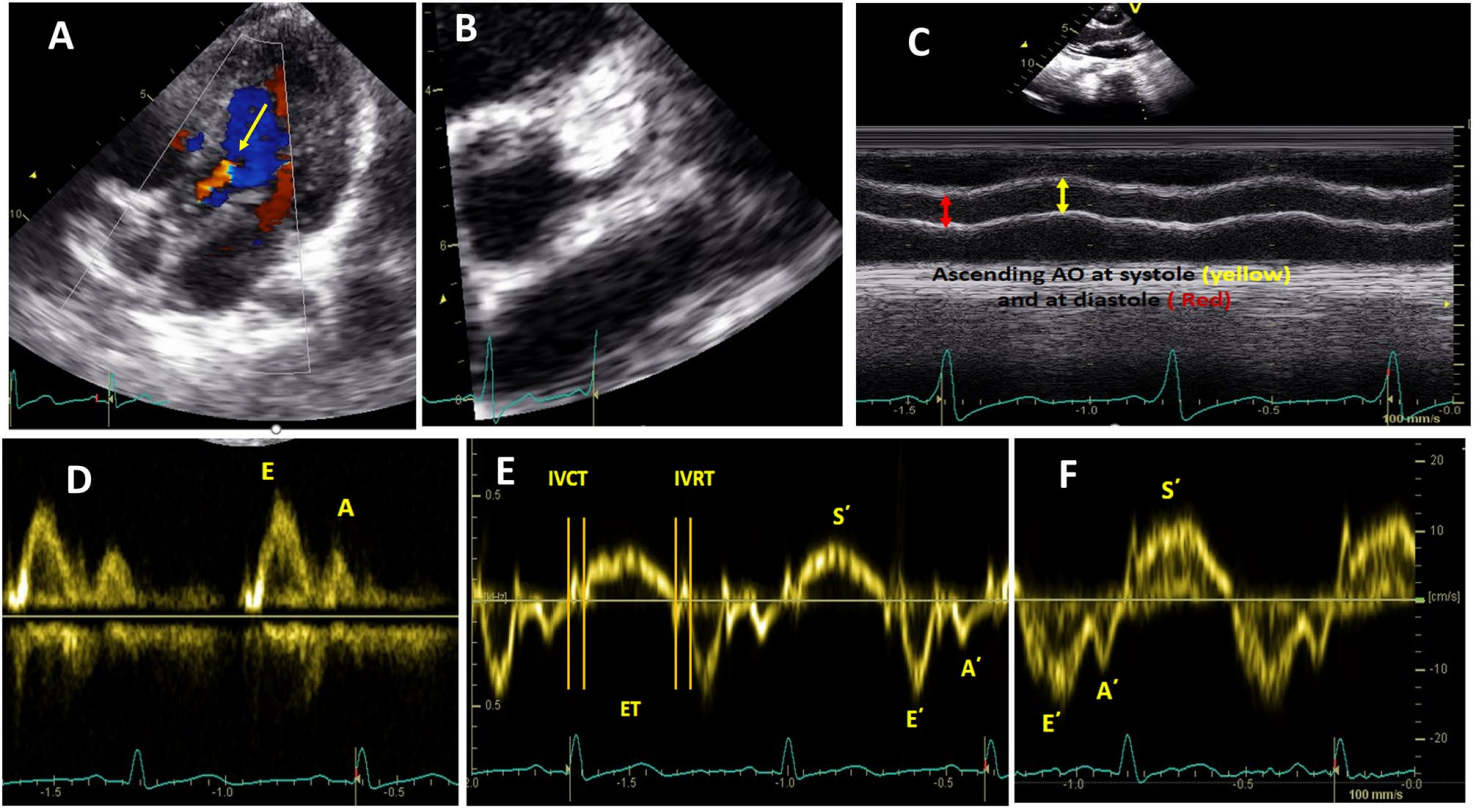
Conventional echocardiography and TDI done for the studied patients. A Apical five-chamber view with color Doppler showing mild aortic insufficiency (yellow arrow) in one patient with homozygous FH. B Parasternal short-axis view with zoom on left coronary artery showing left main coronary artery ectasia. C Calculation of ascending aorta strain using M-mode at ascending aorta in the parasternal long-axis view, then measuring systolic (yellow arrows) and diastolic (red arrows) aortic dimensions. D Pulsed Doppler at mitral inflow showing early (E) and late (A) mitral inflow velocities. E Pulsed wave TDI at the basal part of the LV lateral wall showing myocardial velocities and time intervals. F Pulsed wave TDI at the basal part of the RV lateral wall showing myocardial velocities. AO aorta, ET ejection time, FH familial hypercholesterolemia, IVCT isovolumic contraction time, IVRT isovolumic relaxation time, LV left ventricle, RV right ventricle, TDI tissue Doppler imaging
